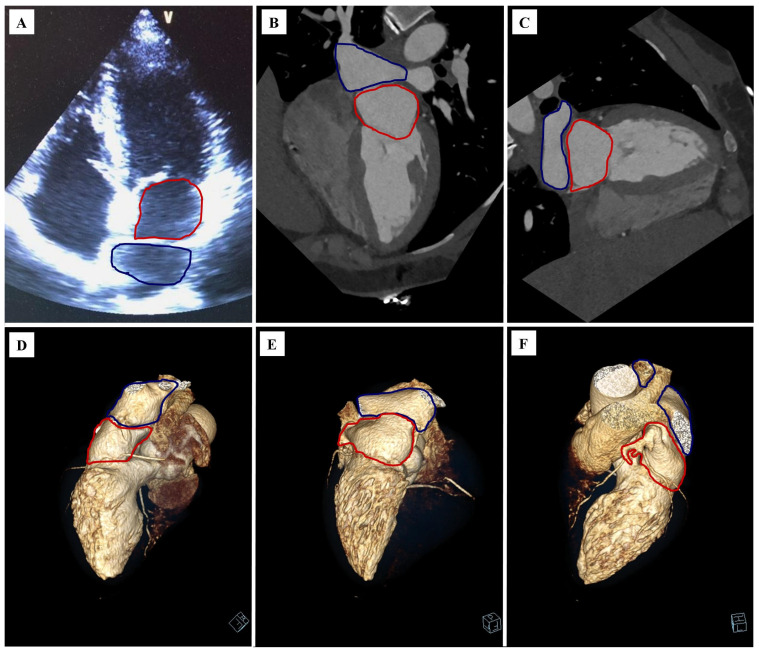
The cor triatriatum sinister. The proper atrium is marked with a red outline, while the accessory atrium is marked with a blue outline: (A) echocardiography, long axis, four-chamber projection; (B) cardiac computer tomography angiography (CCTA), multiplanar reconstruction (MPR), long axis, four-chamber projection; (C) CCTA, MPR reconstruction, long axis, two-chamber projection; (D) CCTA, volume rendering technique (VRT) reconstruction, right side view; (E) CCTA, VRT reconstruction, posterior view; (F) CCTA, VRT reconstruction, left side view.
